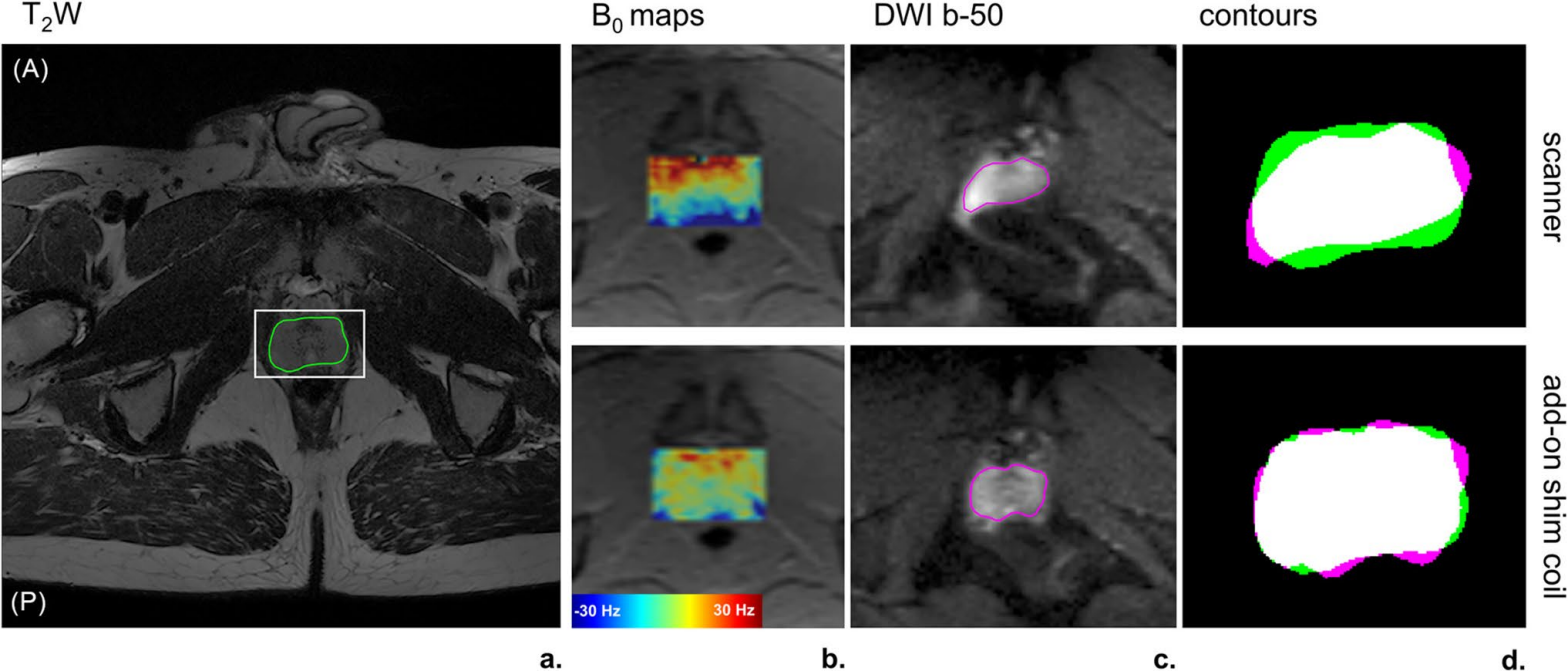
DWI analysis of an example slice in a subject (V3) using both conventional shimming (top) and the add-on shim coil (bottom) approaches a Transversal T2W image as an anatomical reference with the prostate outlined in green and the selected shimming adjustment volume (white frame) b B_0_ maps c b-50 EPI images with the prostate outlined in purple d b-1400 EPI images e resulting overlaid prostate segmentations of T2W contour (green) and b-50 DWI contour (purple) (scanner DSC = 0.87; add-on shim coil DSC = 0.94)
